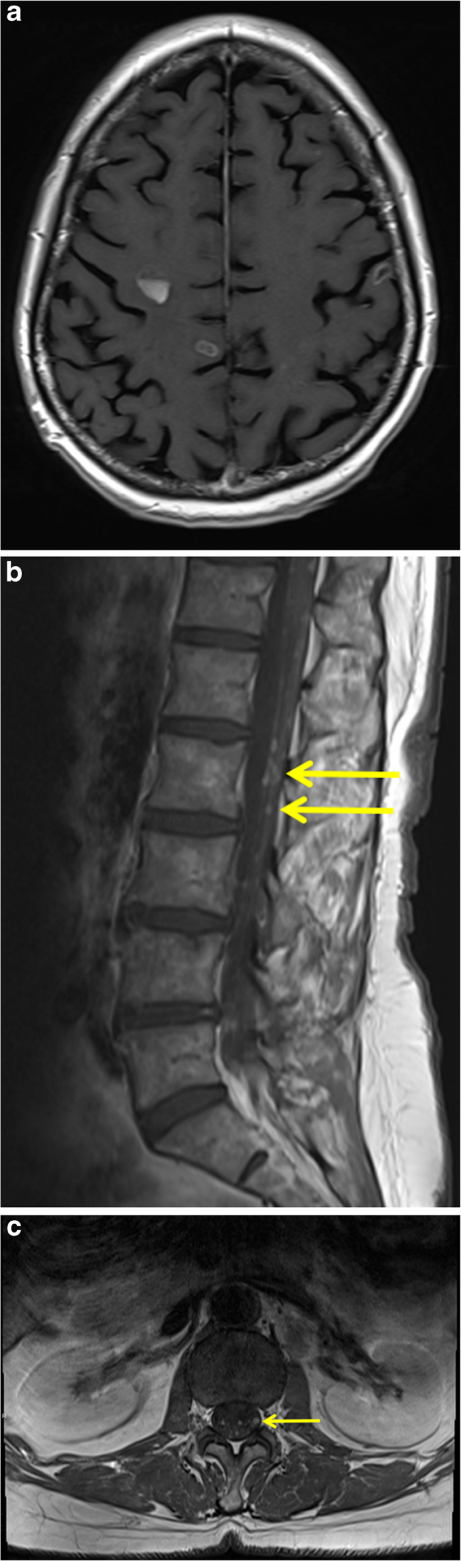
Intradural extramedullary metastases in a 54-year-old woman with non-small cell lung cancer. Axial T1-weighted image of the brain after intravenous gadolinium contrast administration (a) shows presence of multiple ring-enhancing parenchymal lesions consistent with cerebral metastases. Sagittal (b) and axial (c) T1-weighted MR images of the lumbar spine (after intravenous gadolinium contrast administration) demonstrate multiple enhancing tumour nodules (*yellow arrows*) along the lumbar spinal cord and cauda equina indicating leptomeningeal metastatic disease
